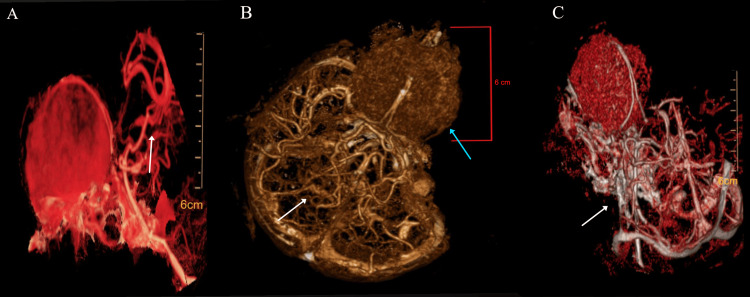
CTA confirmed a vascularized lesion in the olfactory groove region suggestive of a meningioma. (A) Volume-rendered CTA demonstrates a hypervascular, extra-axial lesion located in the olfactory groove region, with arterial supply predominantly arising from the bilateral anterior ethmoidal arteries (branches of the ophthalmic arteries), as indicated by the white arrow. Additional arterial contribution from the ACA, including frontopolar and orbitofrontal branches, is also visualized. These vascular features are suggestive of a meningioma. (B) Three-dimensional volumetric reconstruction highlights the solid tumor component (blue arrow), measuring approximately 6 cm, and the dense cortical vascular network (white arrow), corresponding to feeding branches from the anterior cerebral circulation and ethmoidal arteries. (C) Combined volume-rendered CTA shows prominent intratumoral enhancement, with clearly identifiable arterial feeders and early draining veins (white arrow). Venous outflow is observed through superficial frontal cortical veins, with drainage toward the superior sagittal sinus, underscoring the lesion’s hypervascular nature and its relevance for surgical planning. CTA, computed tomography angiography; ACA, anterior cerebral artery
